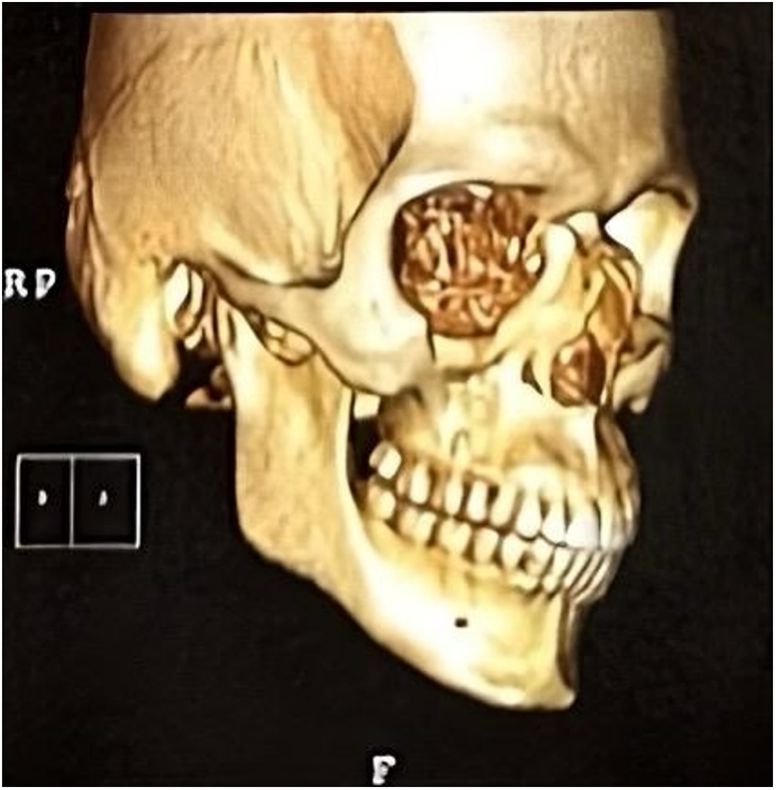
Computed tomography (CT) scan showing isolated medial and inferior orbital wall fractures.
